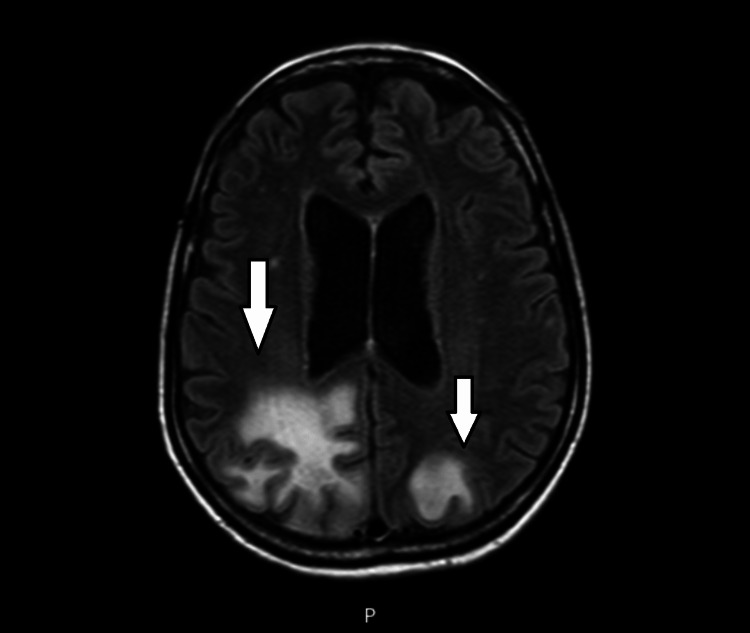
Cranial magnetic resonance imaging (MRI) showing biparietal intra-axial white substance lesions, hyperintense in T2
